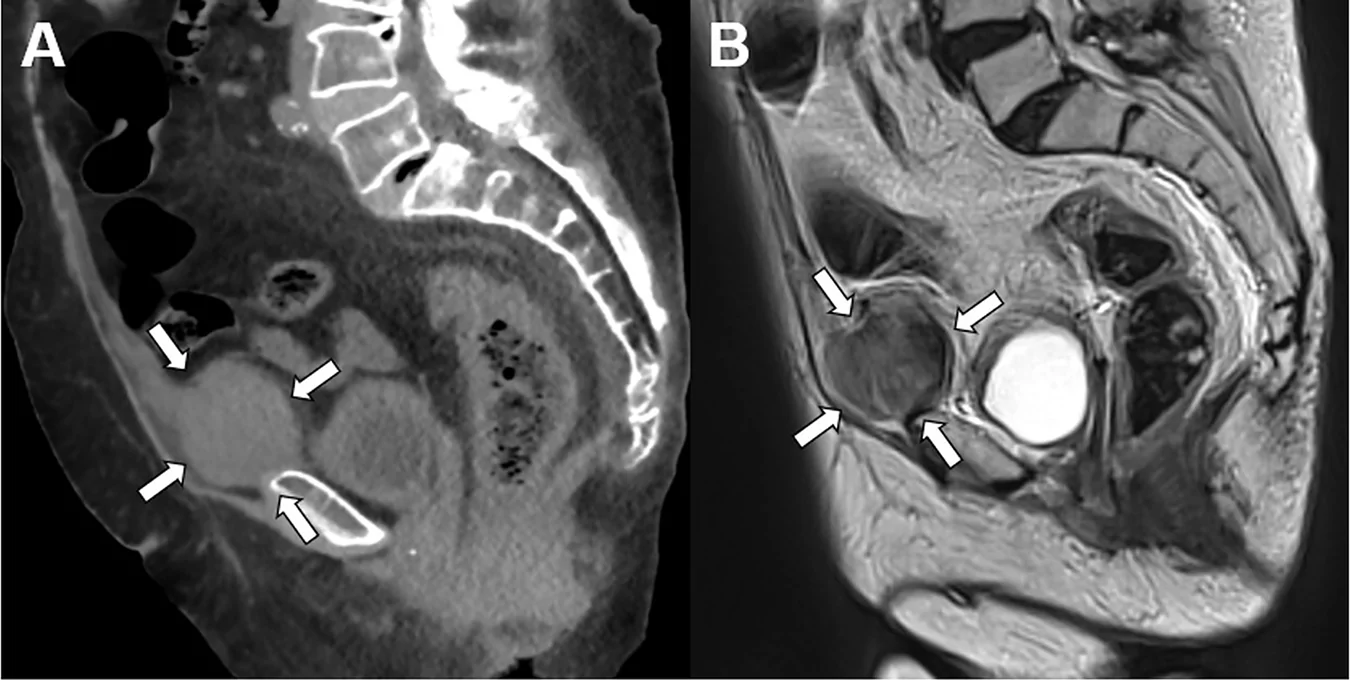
Biopsy-proven malignant glomus tumor of the space of Retzius in an 84-year-old woman. Sagittal unenhanced CT (A) and sagittal T2-weighted MR image (B) demonstrate a well-circumscribed, spherical mass in the space of Retzius (arrows). Intravenous contrast material was not administered in each examination due to impaired renal function
